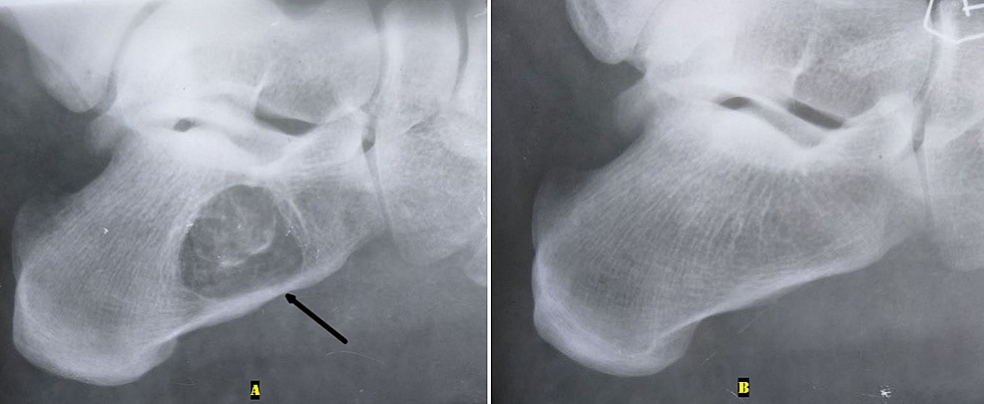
Plain radiograph of both ankles' lateral view A: A lytic lesion was seen in the body of the right intact calcaneum with mild sclerotic margins (black arrow). B: A normal left calcaneum.
